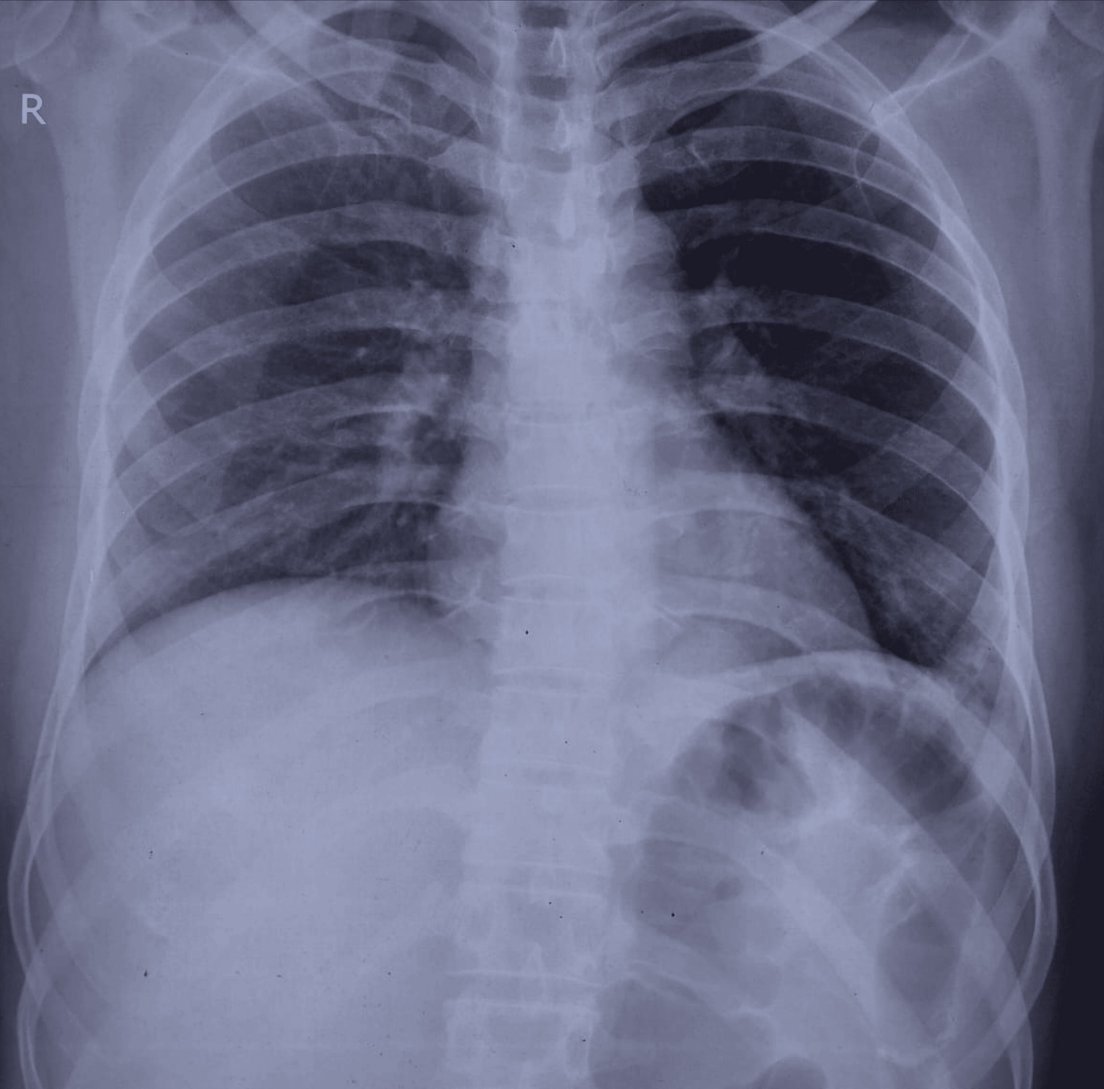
Chest radiograph at presentation. A plain chest radiograph obtained during the initial evaluation demonstrated no clear radiographic evidence of active pulmonary tuberculosis.
